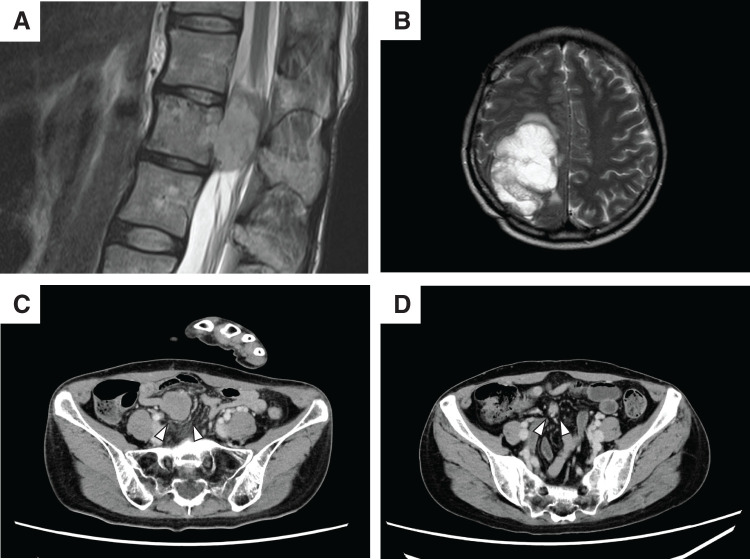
Imaging studies. (A) T2-weighted MRI demonstrating an osteolytic lesion in the 1st lumbar vertebra. (B) T2-weighted MRI demonstrating a metastasis with high signal intensity in the right parieto-occipital lobe, which developed 3 years after treatment of the primary lesion. (C) Contrast-enhanced abdominal CT showing mesenteric lymph node metastasis (white arrowheads), which developed simultaneously with the brain metastasis. (D) The mesenteric metastasis (white arrowheads) markedly shrank (to 30 mm in size) after 6 cycles of IFM therapy. IFM, ifosfamide
